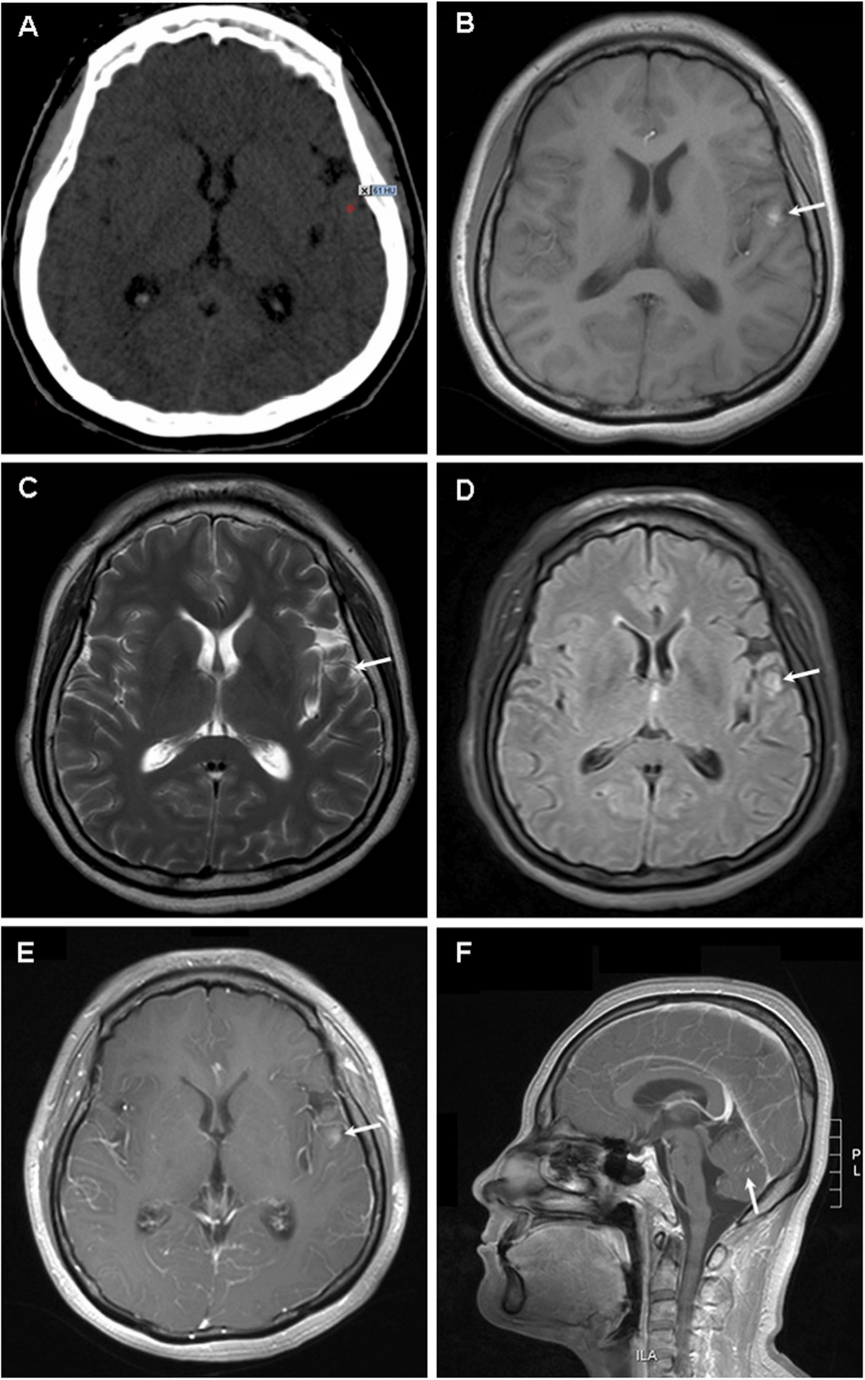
Imaging findings for brain. (A) Computed tomography image showing an isodense or slightly hyperdense lesion in the left temporal lobe (CT value: 45 to 61). Magnetic resonance image showing a 6-mm abnormal signal in the left temporal lobe, which was hyperintense on T1-weighted images (B), hypointense on T2-weighted images (C), slightly hyperintense on fluid-attenuated inversion recovery images (D), and mild enhancement with gadolinium contrast (E). Punctate and linear enhancement in the sulci and gyri of the cerebellum can be observed (F).
